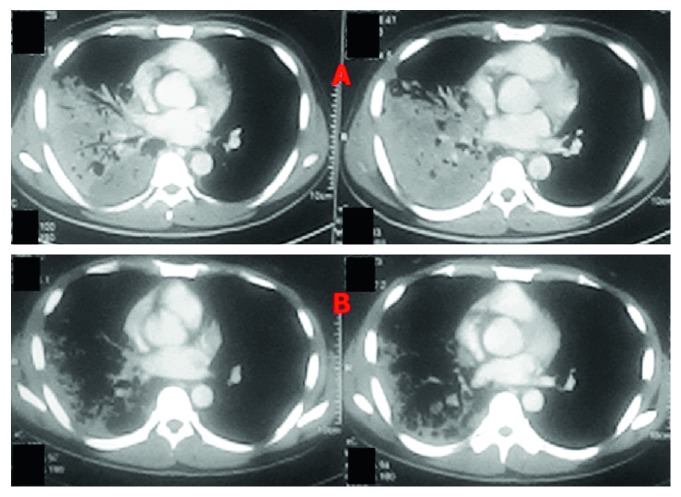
Two chest CT scans were undertaken 14 days apart. (a) The first chest CT showing consolidation at the right lower lobe with small cavities. (b) The second chest CT with significant amelioration of consolidation at the same lobe.
